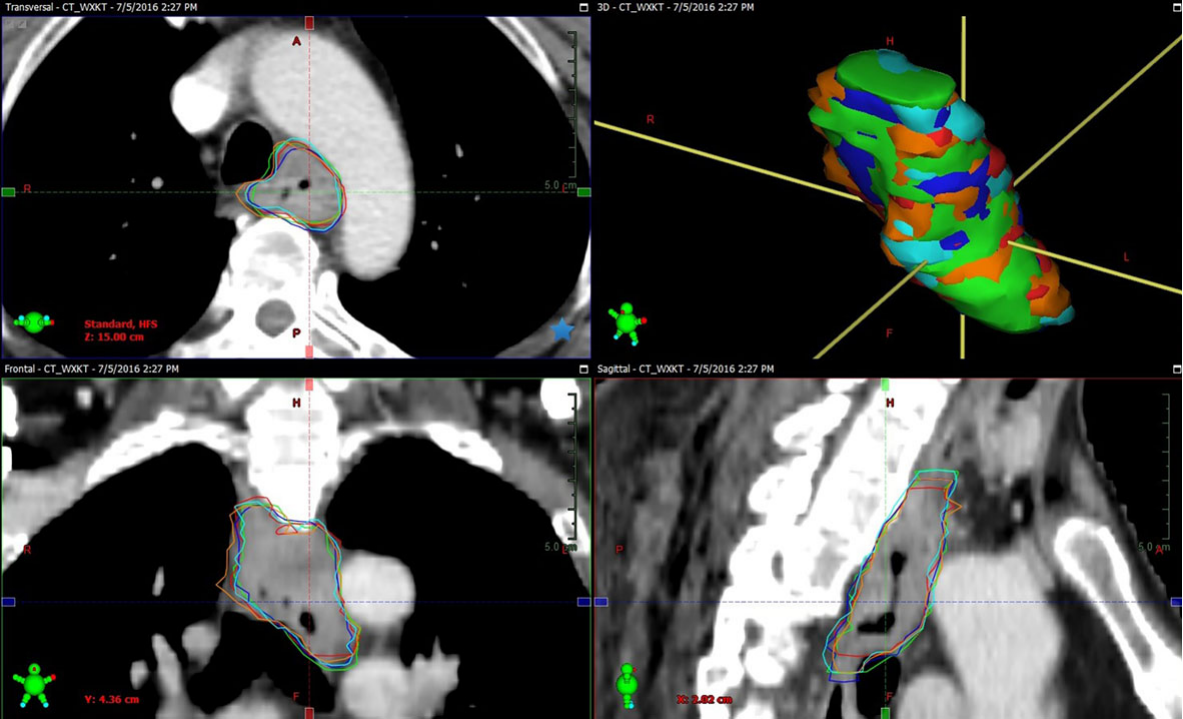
Example of GTVs delineated based on CT combined with endoscopy/EUS, esophagogram, and FDG-PET/CT (GTV_CEXP_) by observer 1 (green segment), observer 2 (red segment), observer 3 (blue segment), observer 4 (orange segment), and observer 5 (cyan segment) in tansversal, frontal, surface, and sagittal planes for one patient (Patient 10). The volume and longitudinal length of GTV_CEXP_ delineated on CT combined with endoscopy/EUS, esophagogram, and FDG-PET/CT exhibiting a good consistency.
